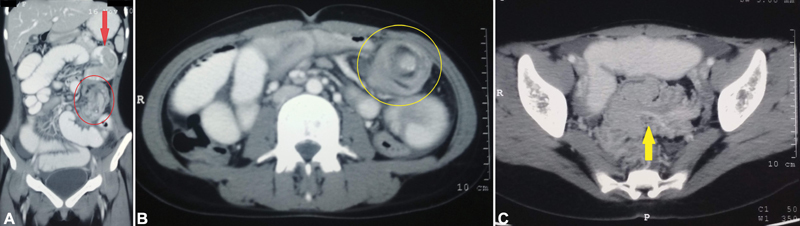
Contrast-enhanced computed tomography of the abdomen showing (
